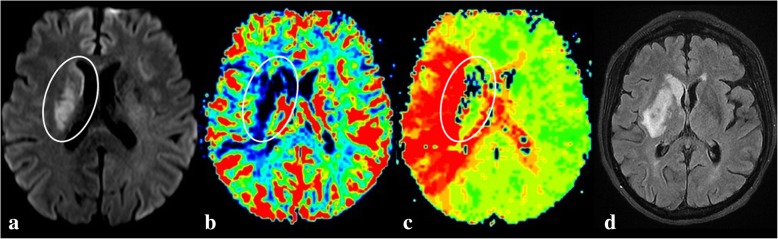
A 67-year woman was admitted with a wake-up stroke. Clinically, she presented with severe right MCA infarct symptoms (NIHSS 14). Since the time of onset of the stroke could not be determined, MRI was performed. The diffusion-weighted trace image (a) shows an infarct core with diffusion restriction in the right basal ganglia. The parametric MR perfusion maps (b, cerebral blood volume (CBV) and c, time to peak (TTP)) show a mismatch indicating a large penumbra, i.e., area of potentially salvageable brain tissue. Subsequently, a successful mechanical thrombectomy was performed and the patient had a substantial recovery (NIHSS 3). Follow-up MRI on day 3 after treatment (d) shows that the core infarct remains limited within the right basal ganglia with sparing of the insular, frontal, and parietal cortex
